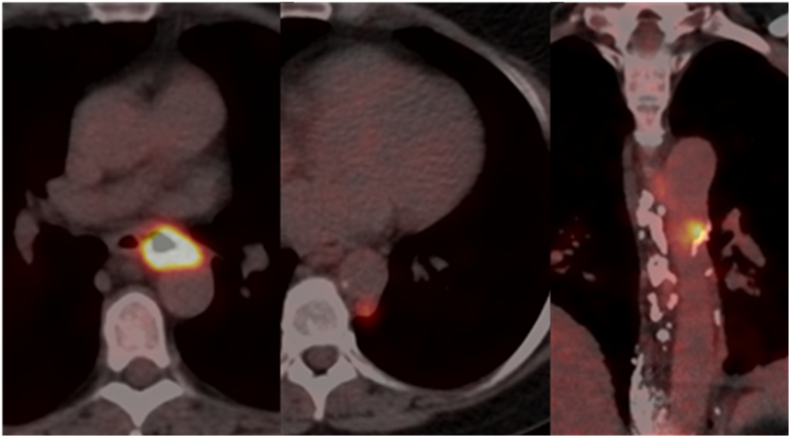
A ^18^F-FDG PET/CT scan of a 57-year-old female with a cT4bN2M1 mid-esophageal squamous cell carcinoma with ingrowth in the aorta and left para-aortic lymph node metastases.
